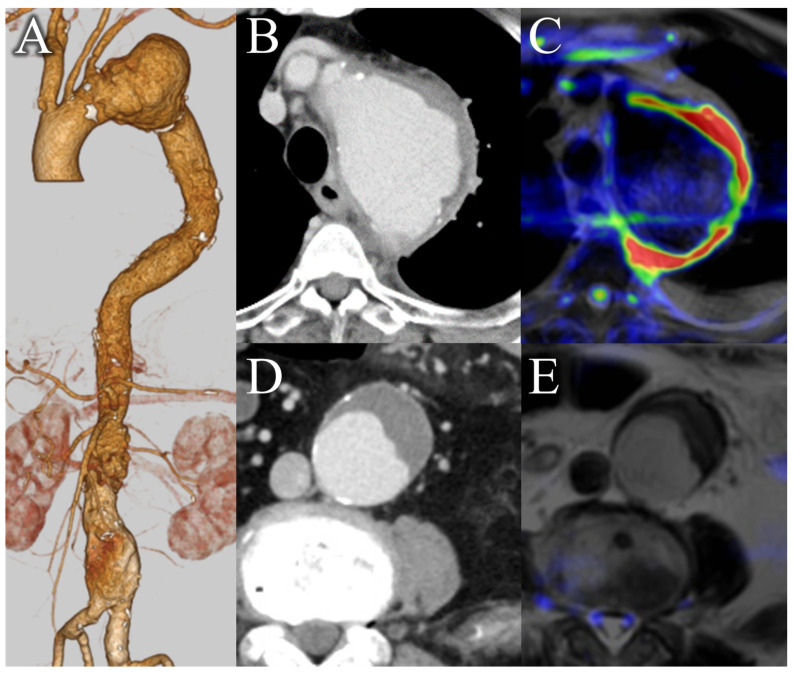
Identifying the extent of infection using DWIBS. A case of aortic aneurysms in both the thoracic and abdominal regions. (A) A 3D reconstruction using CE-CT, (B) Axial CE-CT of the thorax, (C) axial thoracic DWIBS (b = 800 s/mm^2^) fused with T2-weighted imaging, (D) axial CE-CT of the abdomen, and (E) axial abdominal DWIBS fused with T2-weighted imaging. In the fused images (C,E), the blending ratio of DWIBS to T2-weighted images was adjusted to ensure accurate localization. In (B), mild enhancement of the soft-tissue shadow around the aorta, along with a mural thrombus, is noted; the DWIBS image in (C) shows high signal intensity in the same area. In (D), no soft-tissue shadow is visible, although a large mural thrombus is present. In (E), no high signal intensity is observed. The findings indicate that the infection is likely confined to the thoracic aortic aneurysm. CT images are displayed with standard soft-tissue window settings. DWIBS signal intensity is displayed in pseudo-color, where red indicates high signal intensity and blue indicates lower signal intensity. DWIBS, diffusion-weighted whole-body imaging with background suppression; CE-CT, contrast-enhanced computed tomography; CT, computed tomography.
